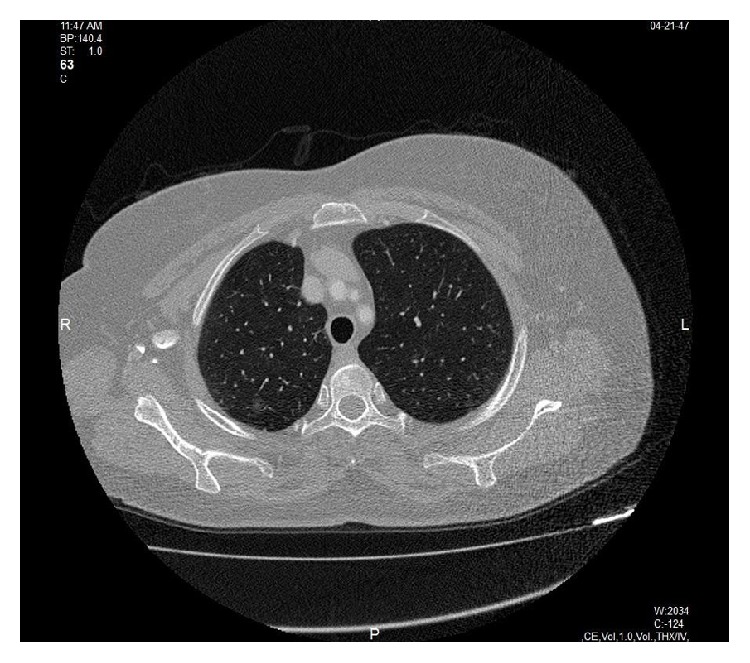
CT examination of the second patient, after 2 years from the lung operation.
